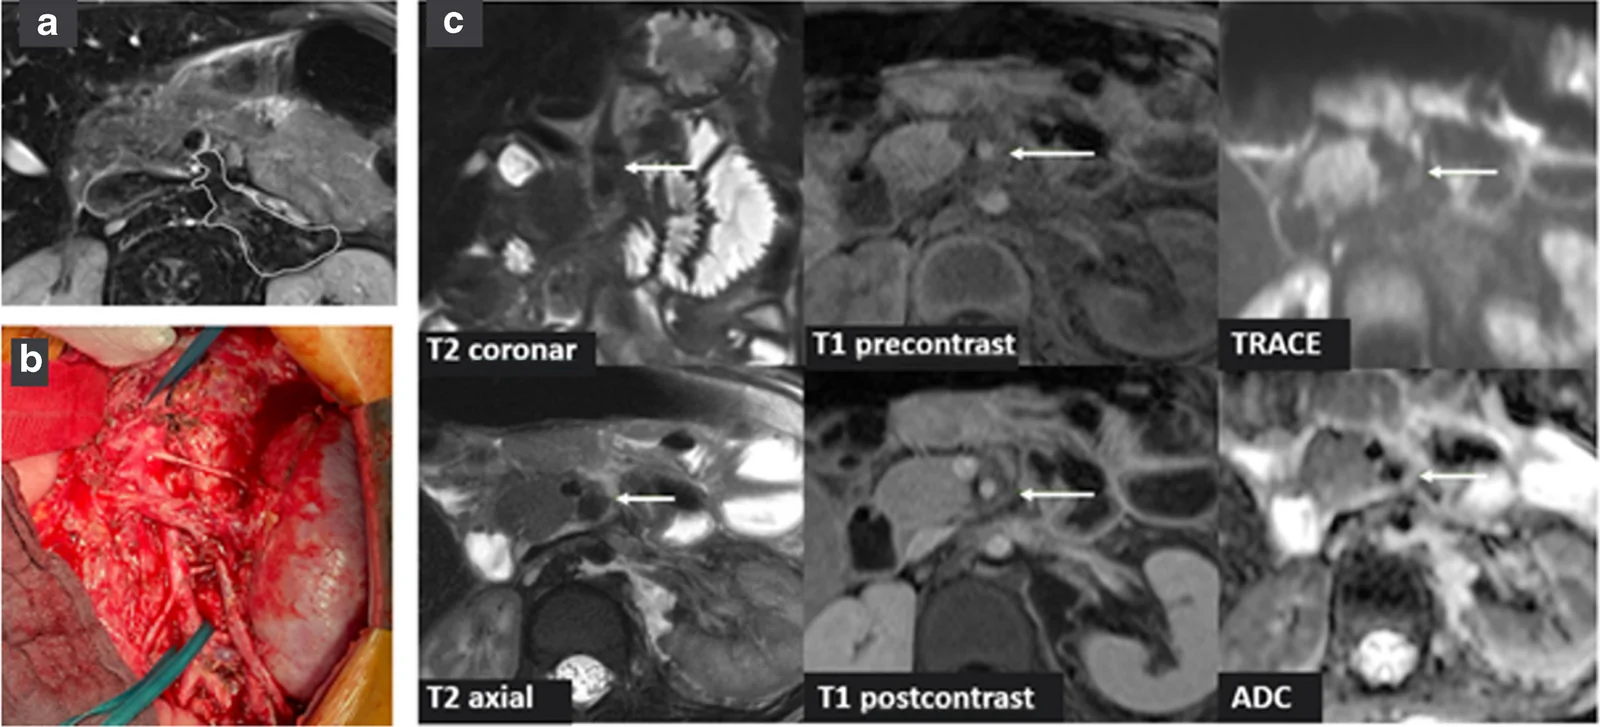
A 5-year-old female patient. a Preoperative T2-weighted magnetic resonance imaging shows neuroblastoma (*cursive*) encasing the superior mesenteric artery (*asterisk*). b Intraoperative image demonstrates no visible residual disease. c Early postoperative magnetic resonance imaging shows subtle residual tumor as eccentric tissue thickening around the superior mesenteric artery (*arrows*). This lesion was not identified by either reader and was classified as a false-negative finding
